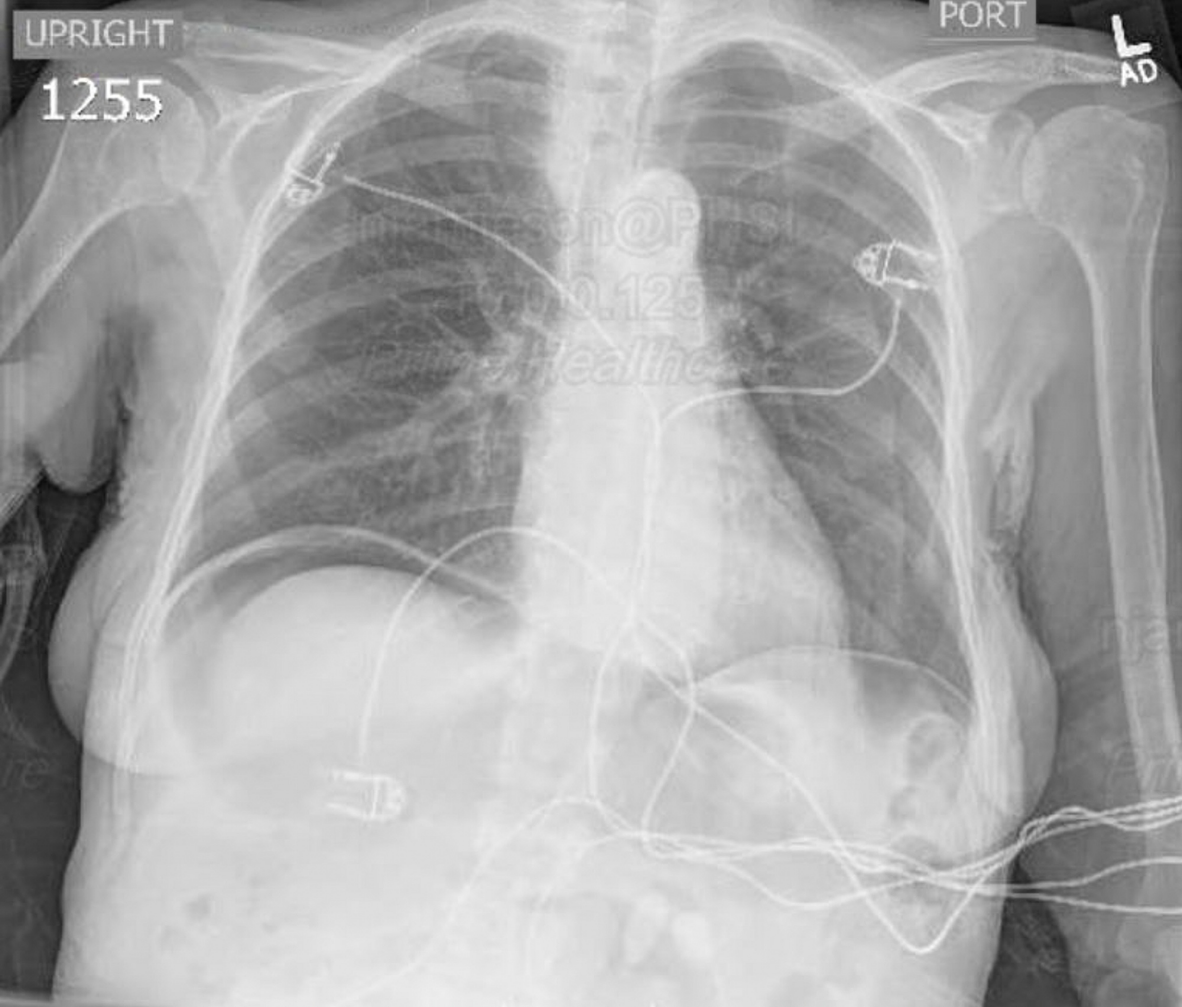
Chest X-ray demonstrating pneumoperitoneum.
